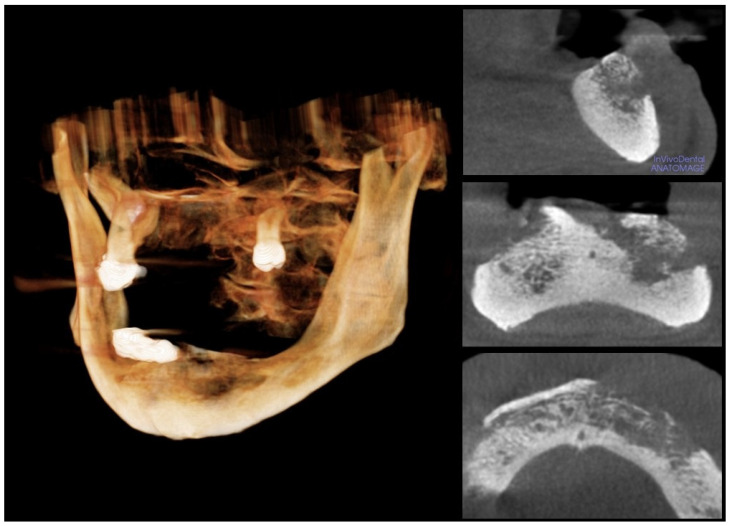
Cone beam computed tomography. The cone beam computed tomography with 3-D reconstruction of the osteolytic area shows the sequestration of the necrotic bone.
